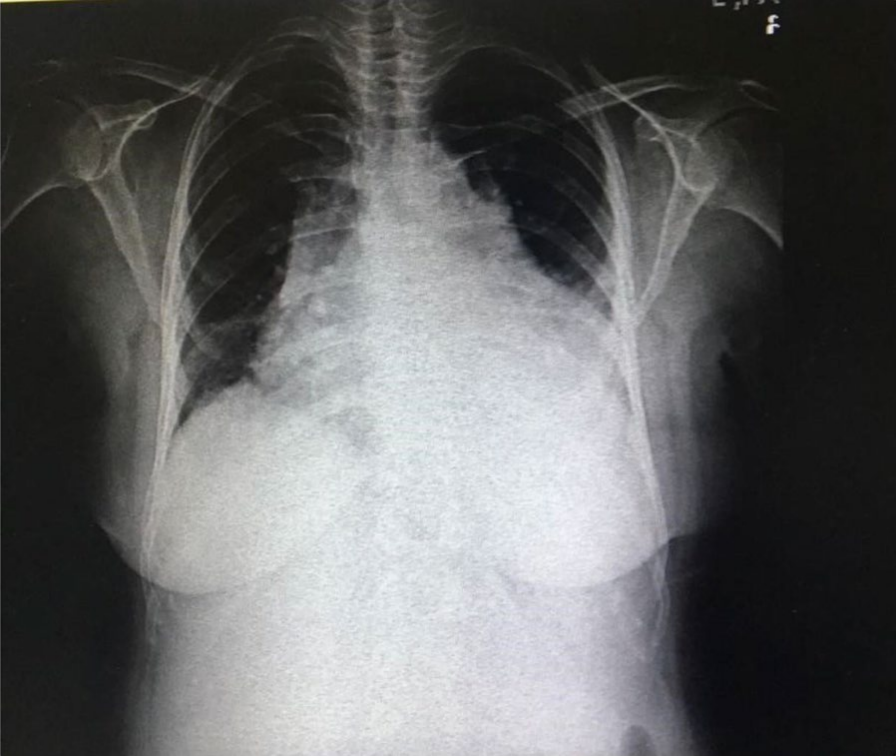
Initial chest radiography of the patient. Chest radiography showing widened mediastinum, cardiomegaly, and left pleural effusion
